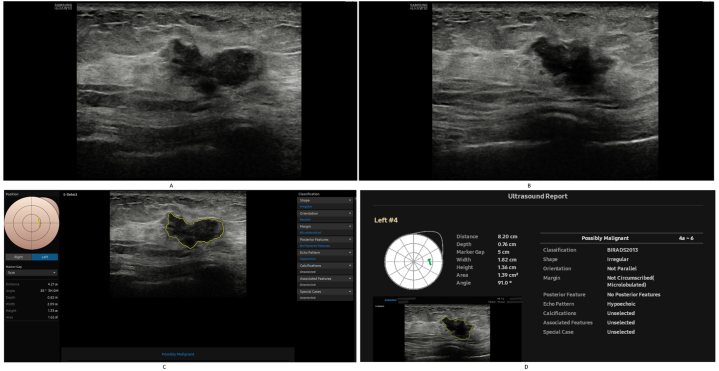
74-year-old woman diagnosed with invasive ductal carcinoma. A-B. Conventional ultrasound showed: on 2D ultrasound, a hypoechoic area is visible in the 2–3 o'clock direction of the upper outer quadrant of the left breast (images from 85° to 91° angle), with an approximate size of 1.4–1.6 cm^2^. The morphology is irregular, and the margin is indistinct. Radiologist classified as BI-RADS 4B. C.CAD description screen (based on 85° angle image): The shape is irregular. The margin is microlobulated. CAD Diagnosis: possibly malignant. D. CAD Report screen (based on 91° angle): The shape is irregular. The orientation is not parallel. The margin is not circumscribed (microlobulated). CAD Diagnosis: possibly malignant. Radiologist reassessed it as category 4C based on multiple CAD reports.
